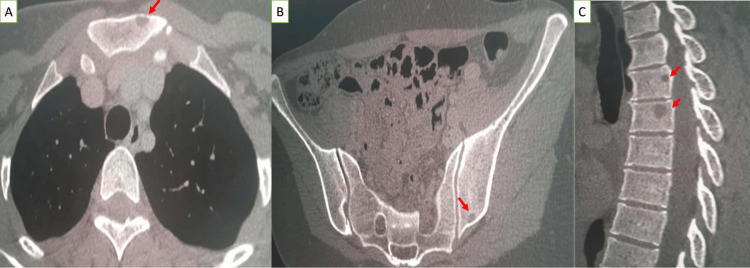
On the bone window of the CT scan, multiple osteolytic lesions without marginal sclerosis were classified as Lodwick Ib visible in the manubium of the sternum (A), the right iliac (B), and the vertebral bodies of the dorsolumbar spine (C).
